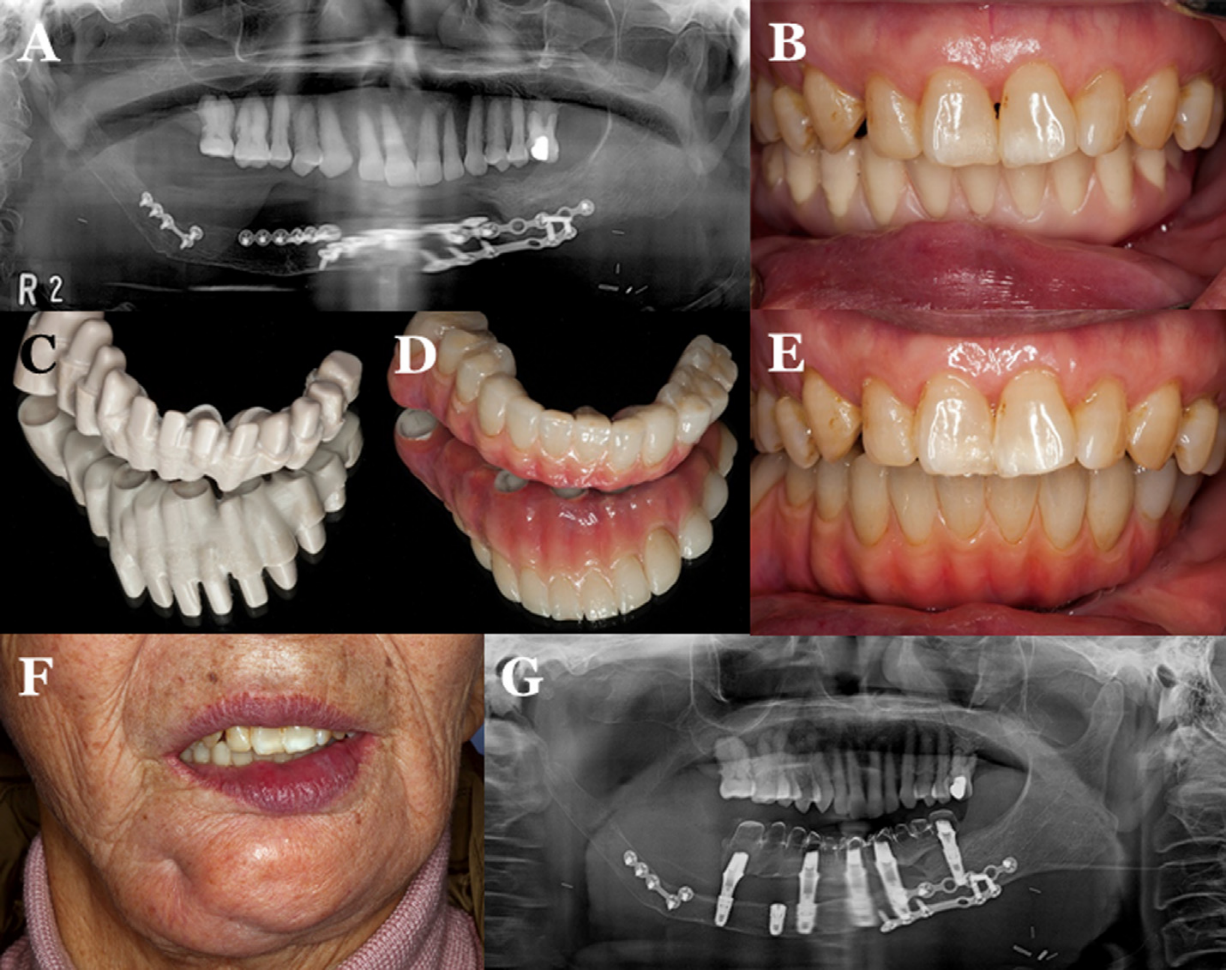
PEKK framework material for rehabilitation of a mandibulectomy patient [63]. (A) Panoramic radiograph of mandible following reconstruction with fibula free flap. (B) Trial denture. (C) PEKK framework fabricated after scanning the completed wax trial denture. (D) Final prosthesis after adding gingiva opaquer and gingiva-colored resins to match the gingiva, and cementing PMMA crowns to the framework. (E) Intraoral view of the prosthesis. (F) Final prosthesis in the patient. (G) Panoramic radiograph at follow up visit.
